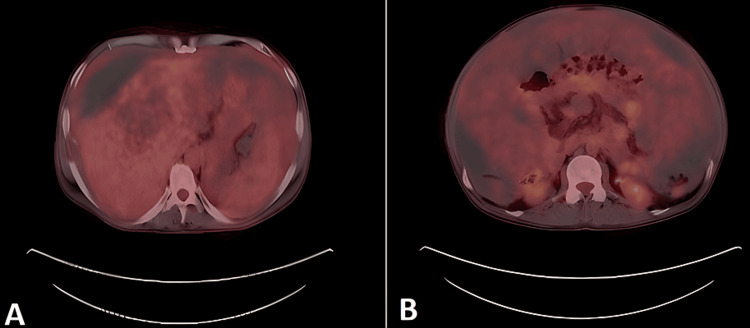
FDG-enhanced PET CT scan axial images of the patient Picture A shows the scalloping of the liver and peritoneal and omental thickening with mild increased uptake surrounding non-FDG-avid mucin. Picture B shows the bowel loops stacked in the mid-line with surrounding non-FDG-avid mucin. FDG: 18F-fluorodeoxyglucose
